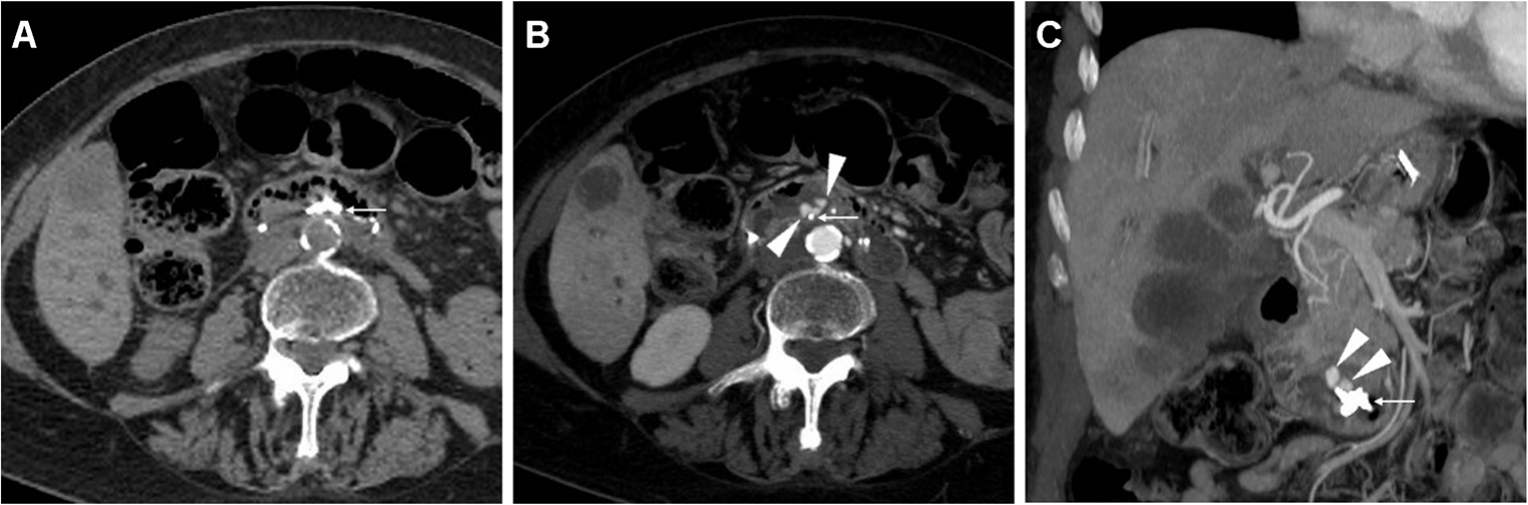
Acute intraluminal bleeding in the duodenum manifesting with acute abdominal pain and impending haemodynamic shock, hours after an unsuccessful endoscopic attempt to relieve the intrahepatic biliary obstruction in 77-year-old female with recently diagnosed infiltrating gallbladder carcinoma. Operative ERCP was interrupted after sphincterotomy because of duodenal haemorrhage, which was treated with epinephrine injection and endoluminal clipping. Comparison of unenhanced (a) and arterial-phase (b, c) CT images detected active contrast extravasation in the duodenal lumen (*arrowheads*) abutting the metallic clips (*thin arrows*), consistent with persistently ongoing arterial haemorrhage. Endovascular therapeutic embolisation of the gastroduodenal artery was necessary to control bleeding
